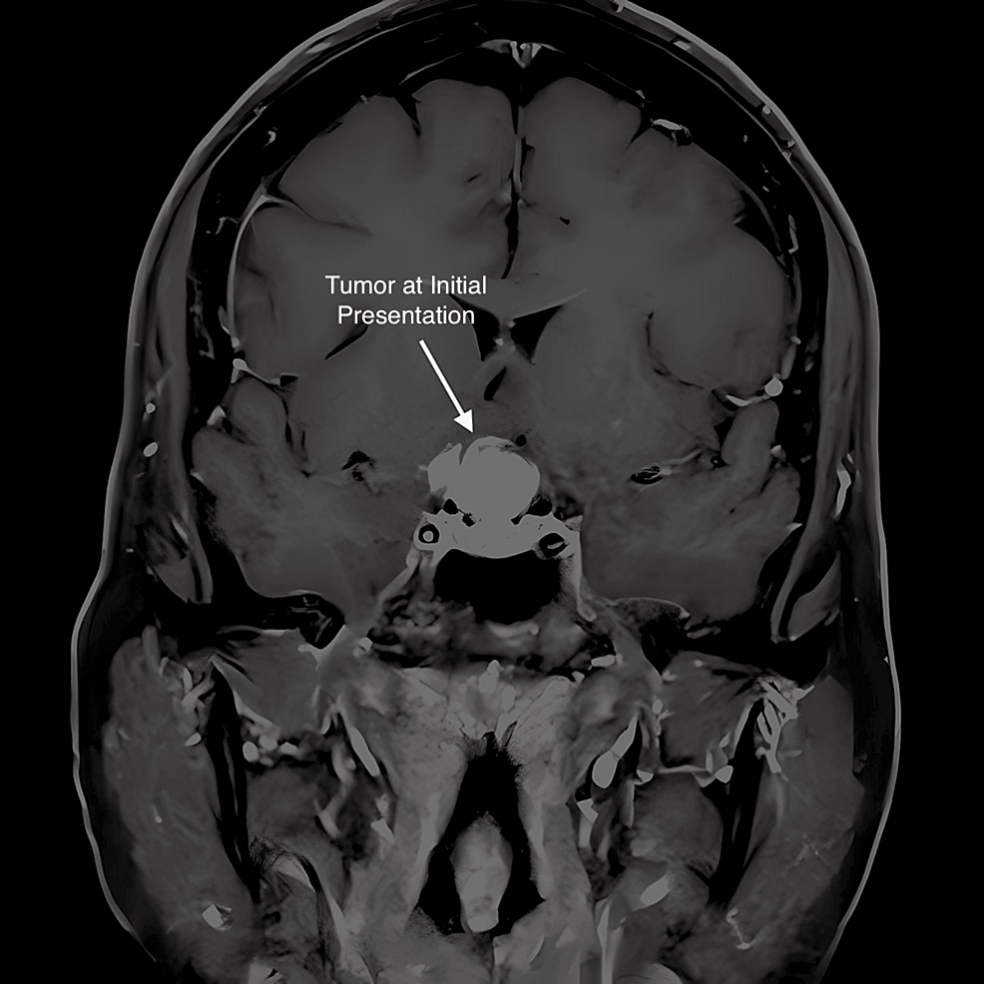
Preoperative mid-coronal MRI with contrast; this shows a 1.8 cm x 1.6 cm x 1.9 cm suprasellar craniopharyngioma with a large cystic component resulting in mass effect on the right prechiasmatic optic nerves and optic chiasm
